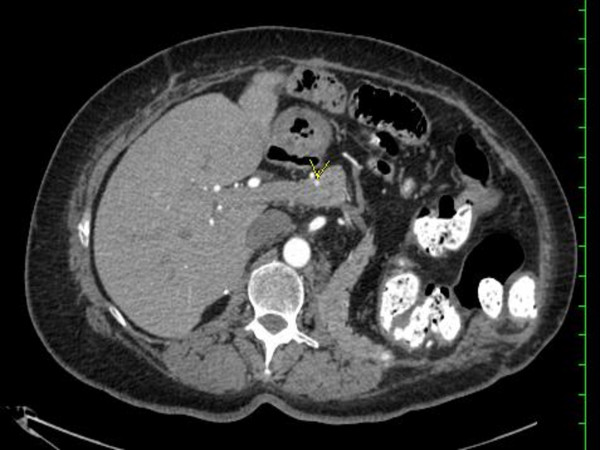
Radiological evaluation. A computed tomography of the chest and abdomen demonstrated multiple small enhancing nodules throughout the pancreas.
